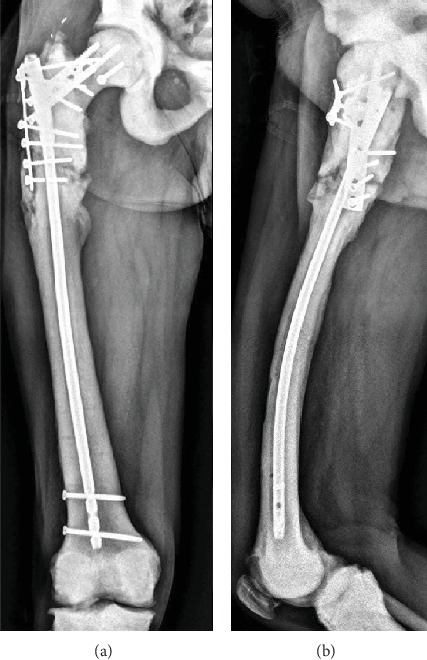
Two-year follow-up. Anteroposterior (a) and lateral (b) radiographs of the femur.
